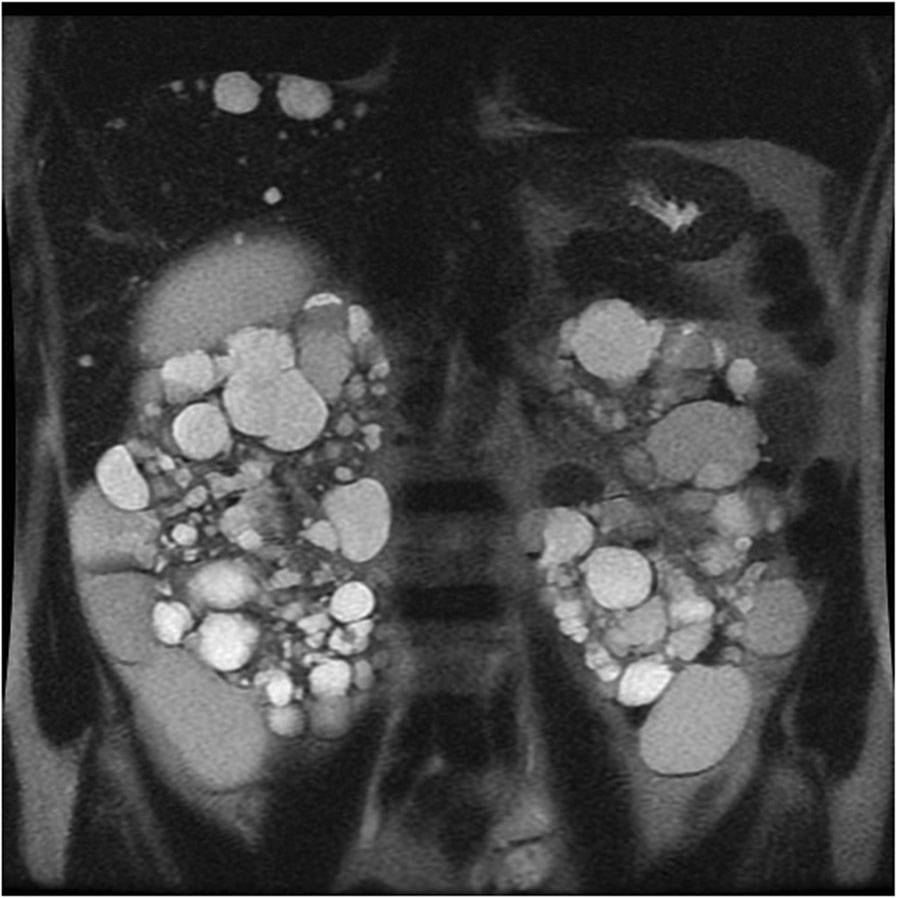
Autosomal dominant polycystic kidney disease. Coronal T2-weighted image shows symmetric enlargement of the kidneys, which contain multiple cysts with variable size
